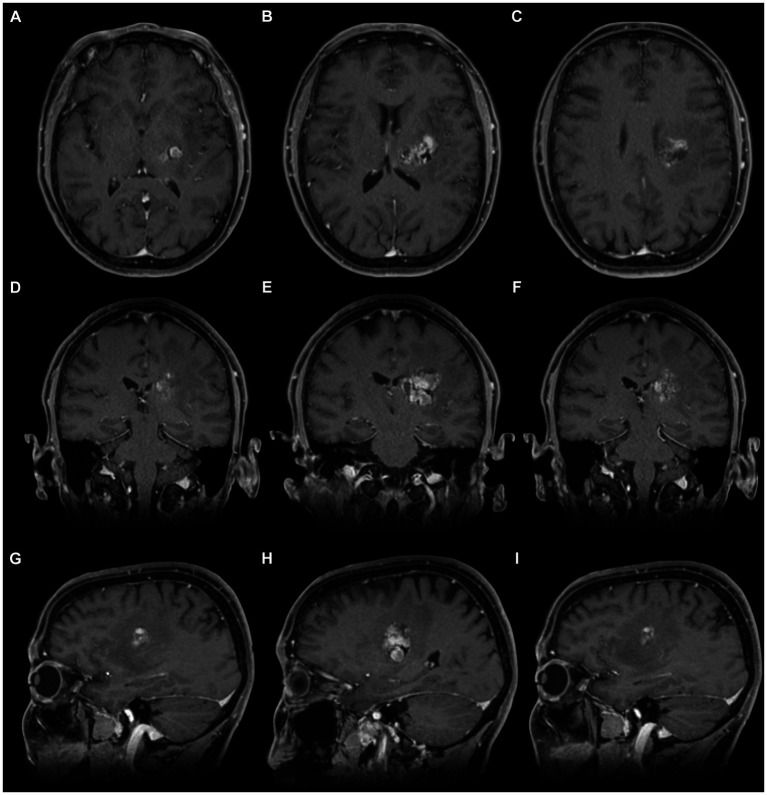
Brain magnetic resonance imaging (T1). (A–C) Axial section. (D–F) Coronal section. (G–I) Sagittal section, where the cavernoma (hypertensity image—heterogeneous) is observed at the level of the posterior limb of the internal capsule. Note its superficial lateral relationship with the posterior aspect of the insula in the sagittal section. The location is posterior to the knee of the internal capsule and the foramen of Monro in the axial section. The arrangement is parallel and inferior to the body of the lateral ventricle in the coronal section.
